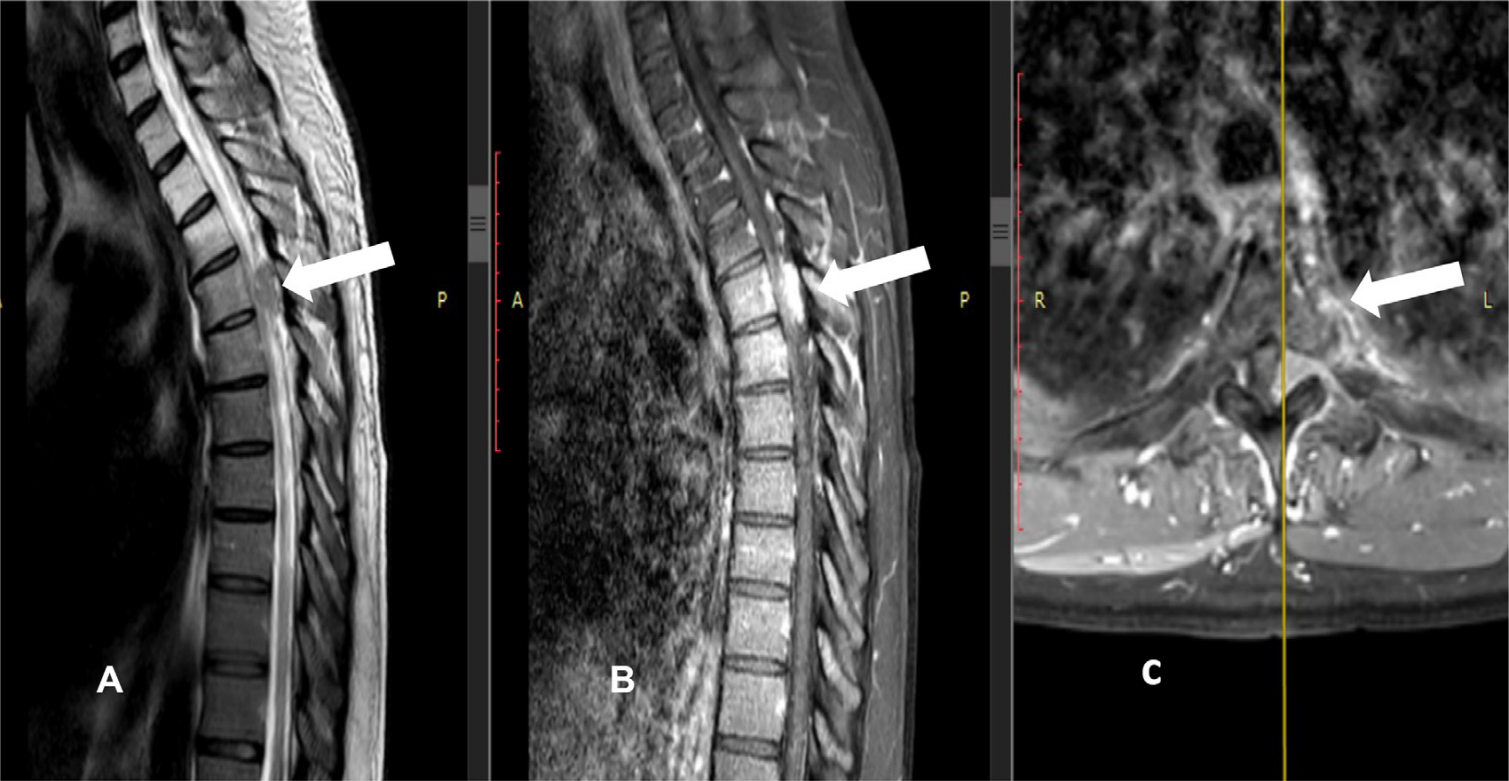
Sagittal T2 weighted (A), T1 weighted MR images following gadolinium administration with fat saturation in sagittal (B) and axial (C) planes show a dural-based lesion at level of T4 vertebra, causing spinal canal stenosis and cord compression (epidural spinal cord compression scale [ESCC] grade 3 cord compression).
